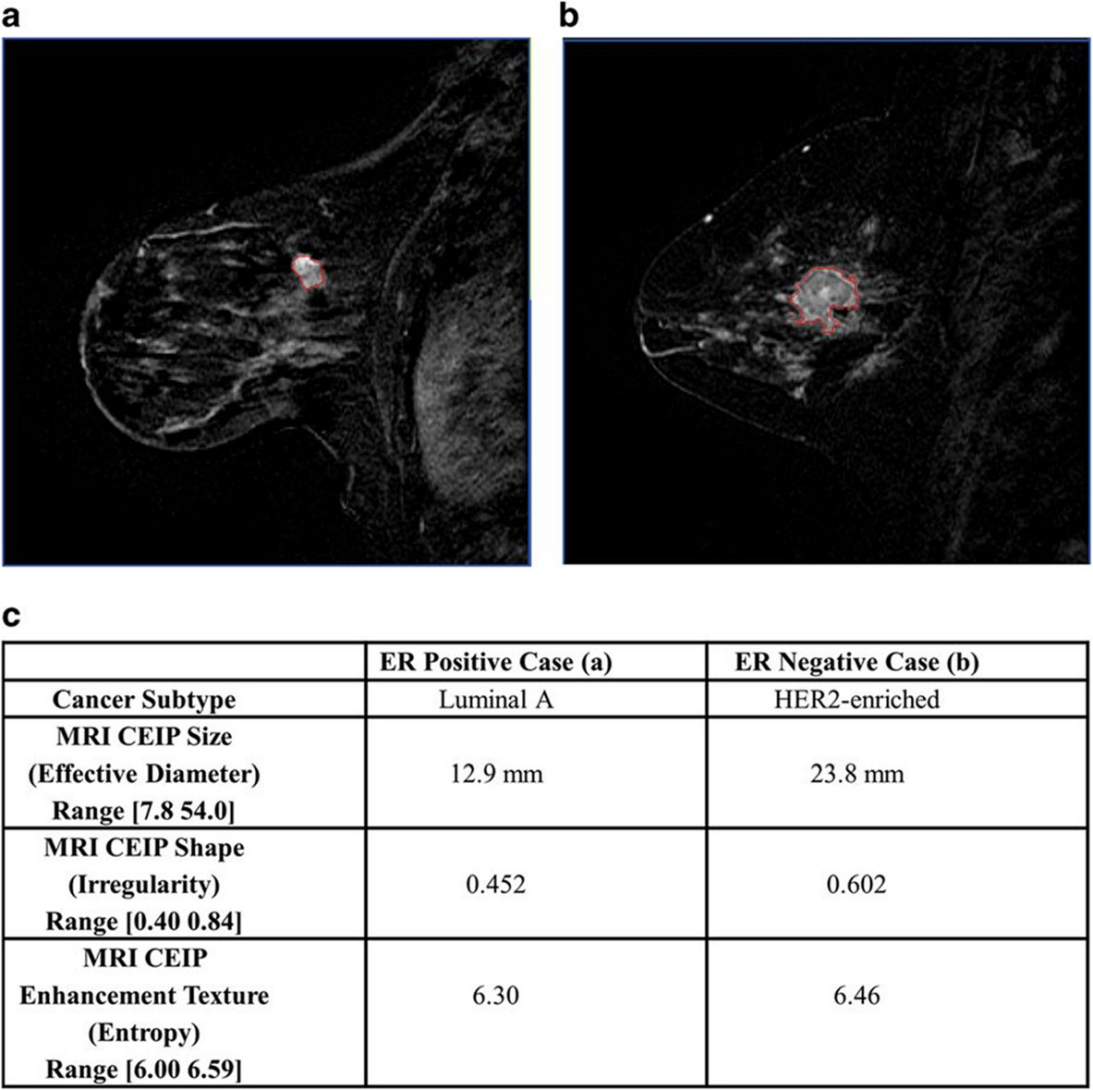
The computer segmentation method in example cases of one estrogen receptor positive tumor and one estrogen receptor negative tumor. The tumor segmentation outlines are shown along with computer-extracted image phenotype (CEIP) values (and ranges) for size, irregularity, and contrast enhancement heterogeneity. Reprinted under a Creative Commons license from: NPJ Breast Cancer. 2016;2. pii: 16012. Epub 2016 May 11. Quantitative MRI radiomics in the prediction of molecular classifications of breast cancer subtypes in the TCGA/TCIA data set. Li H, Zhu Y, Burnside ES, Huang E, Drukker K, Hoadley KA, Fan C, Conzen SD, Zuley M, Net JM, Sutton E, Whitman GJ, Morris E, Perou CM, Ji Y, Giger ML
